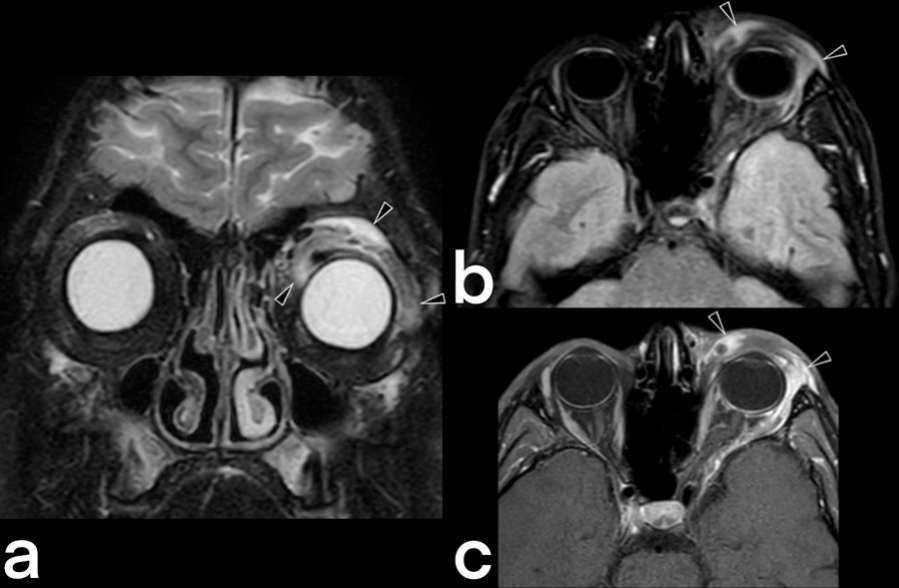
A 19-year-old male patient. T2 weighted coronal (a), axial FLAIR (b), and T1 weighted post-contrast axial (c) images show periorbital involvement (arrowheads)
